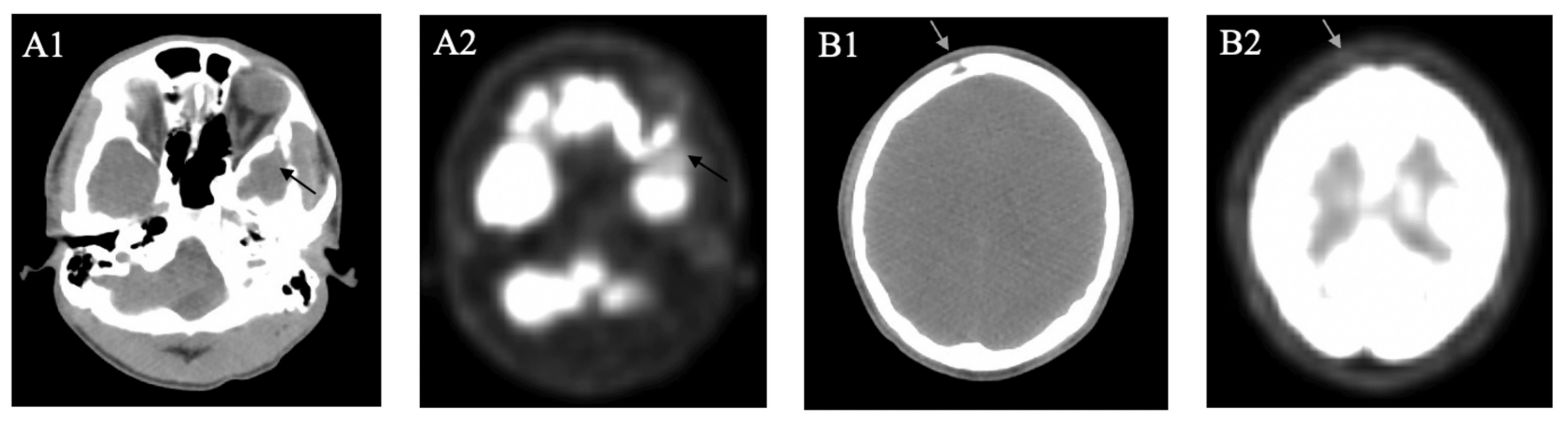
PET/CT findings after three cycles of cladribine. (A1) CT, (A2) PET: Left sphenoid mass size reduction (arrow). (B1) CT, (B2) PET: Interval size increase of the right frontal lytic lesion (arrow). PET/CT, positron emission tomography/computed tomography
